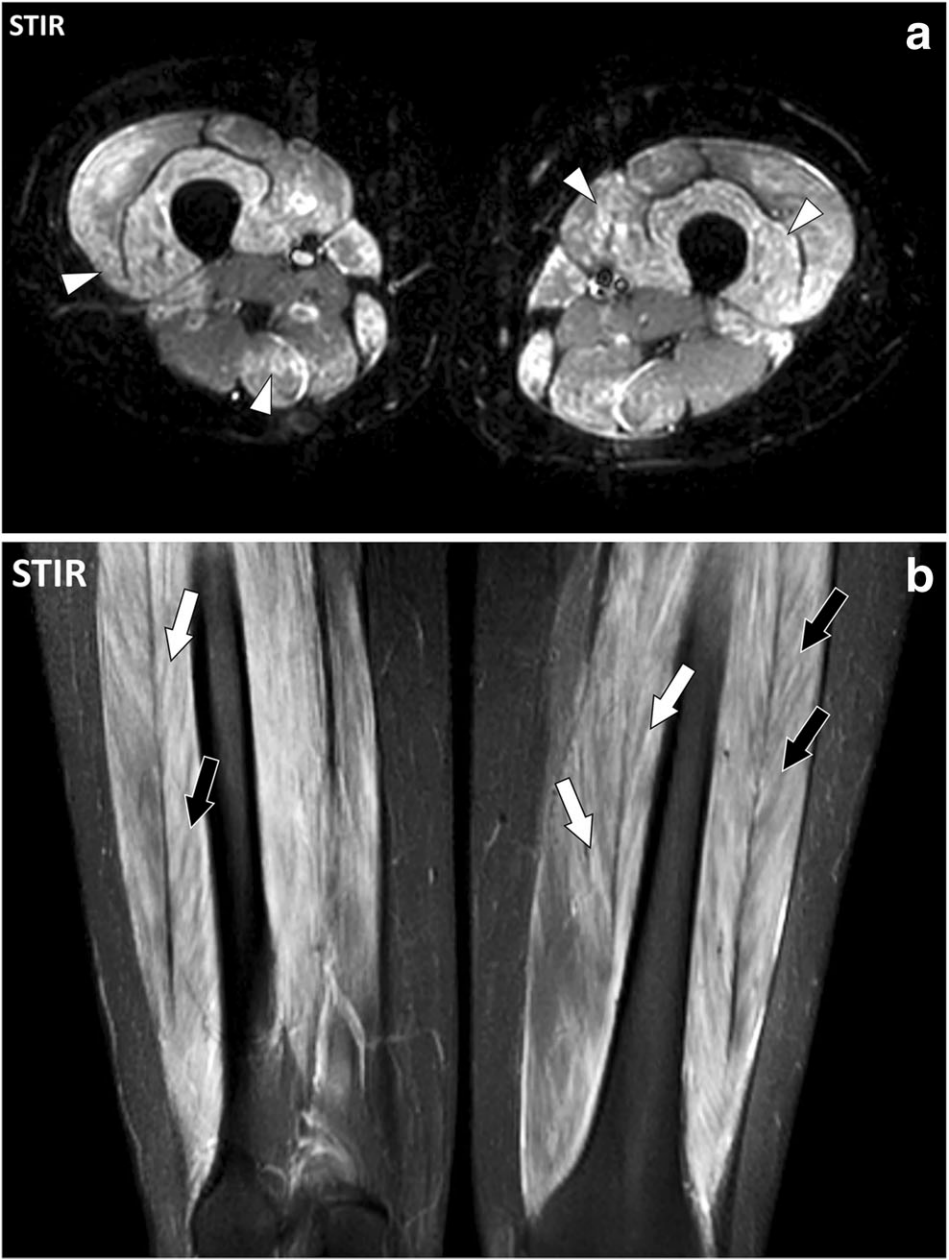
a Axial and (b) coronal STIR images of the thighs of a 16-year-old female with dermatomyositis and anti-TIF1-γ antibodies. MRI demonstrates bilateral symmetrical involvement of the muscles mostly in the quadriceps and gracilis muscles. Blood level of creatine phosphokinase (CPK) was normal. At MRI, involved muscles are heterogenous with a “salt and pepper” pattern in the axial plane (arrowheads) while they maintain a fibrillar organization with fluid signal intensity (white arrows) and low signal intensity (black arrows) striations in the coronal plane
